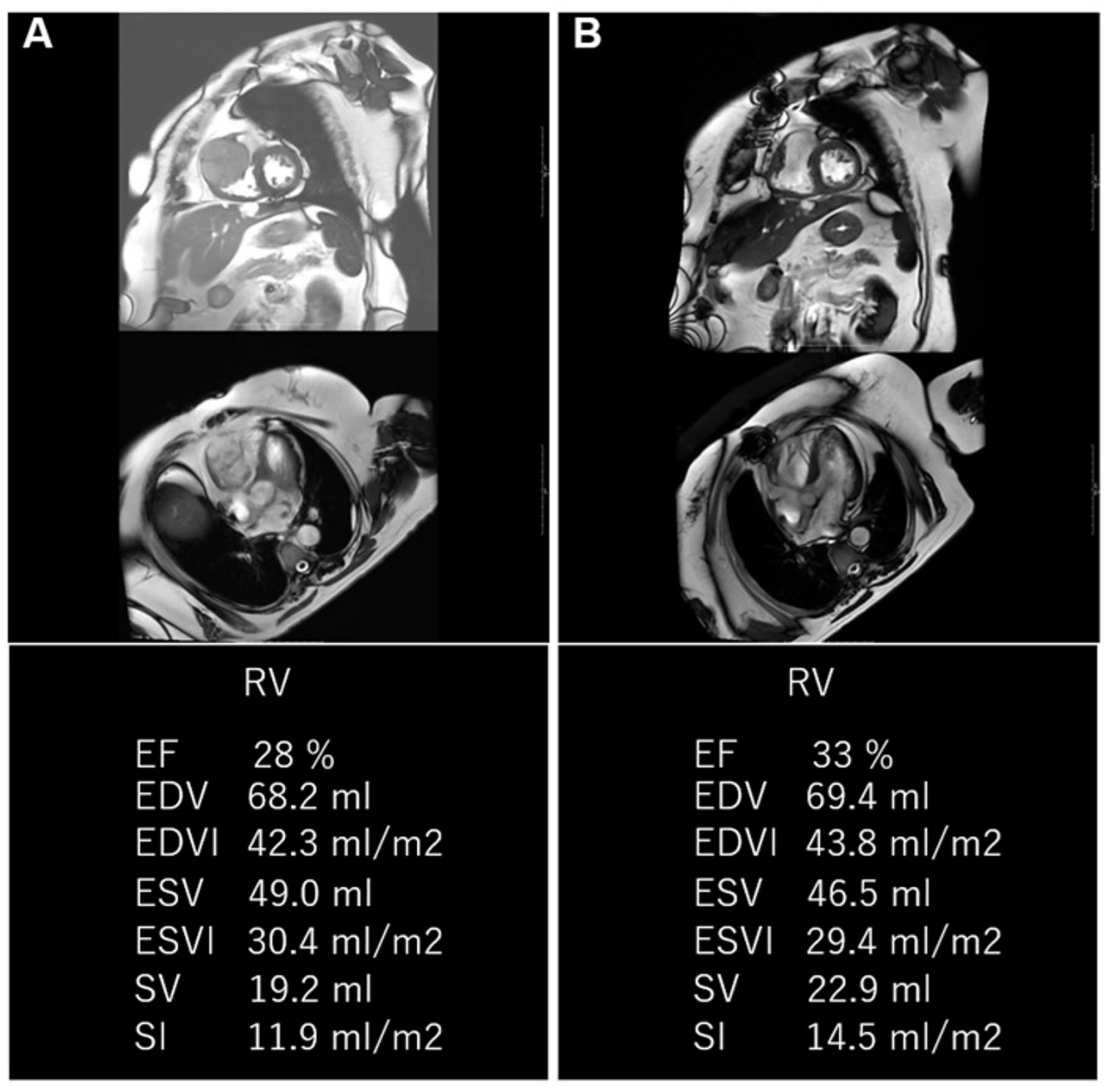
Preoperative and postoperative assessment of right ventricular (RV) size and function using cardiac magnetic resonance imaging (MRI). (a, b) Sagittal and horizontal views of the right and left ventricles obtained before and after surgery, respectively. The lower panels show quantitative parameters of RV function. Postoperative evaluation indicated mild improvement in both end-diastolic volume and contractility. EF, ejection fraction; EDV, end-diastolic volume; EDVI, end-diastolic volume index; ESV, end-systolic volume; ESVI, end-systolic volume index; SV, stroke volume; SVI, stroke index
